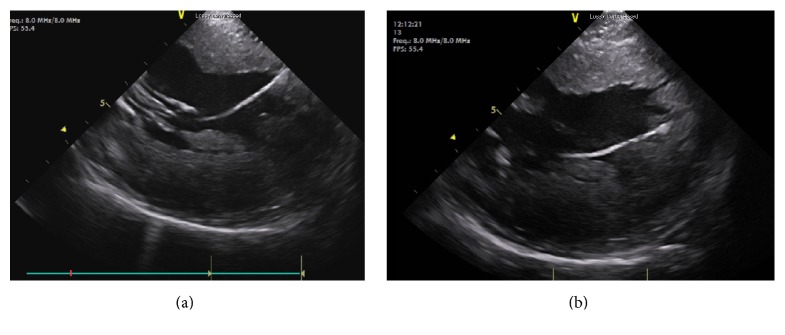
(a) Intracardiac echocardiography showing the tip of the catheter at the anterior side of anterior papillary muscle. (b) Intracardiac echocardiography showing the tip of the catheter at the posterior side of anterior papillary muscle. Ice formation is shown at the tip of cryocatheter during cryoenergy application.
